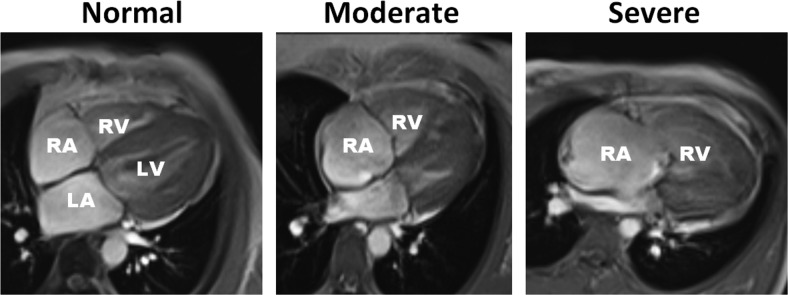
Right ventricular (RV) dilation. Four-chamber cine MR images showing a patient with nondilated RV (left) as well as patients with moderate (middle) and severe (right) RV dilation. Note the large chamber size, which leads to increased RV volume. The right atrium shows corresponding dilation in the provided examples.
